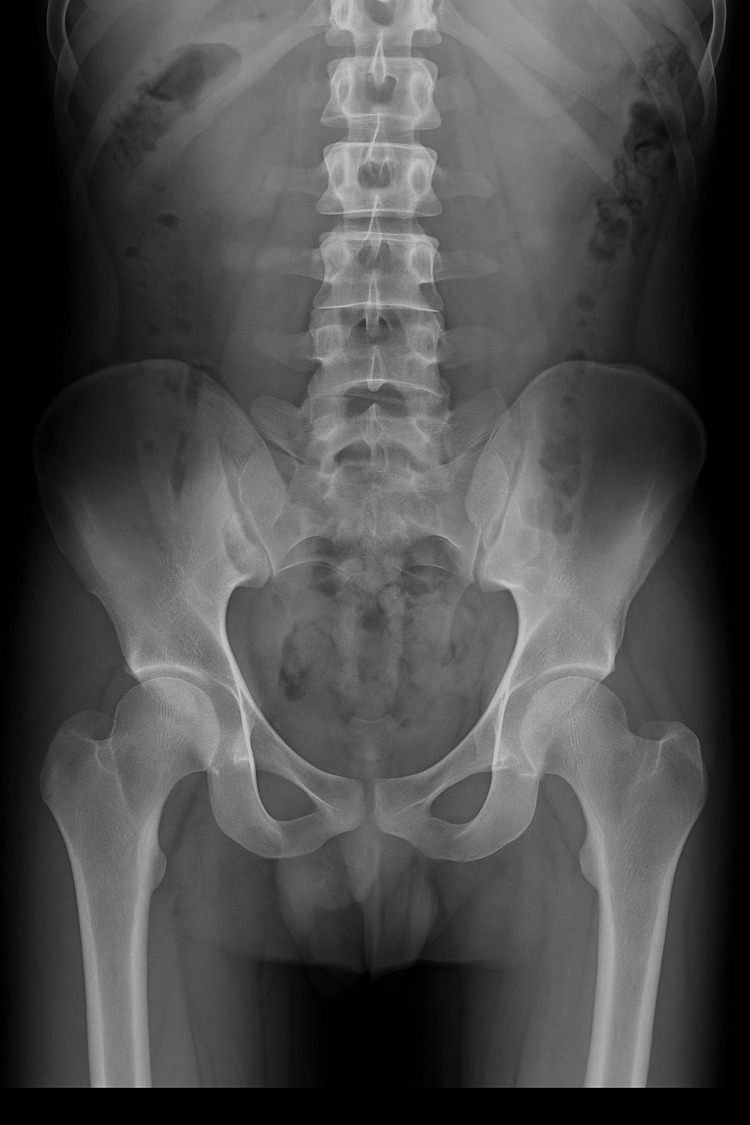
X-ray on the day of presentation No obvious bone pathology is noted.
